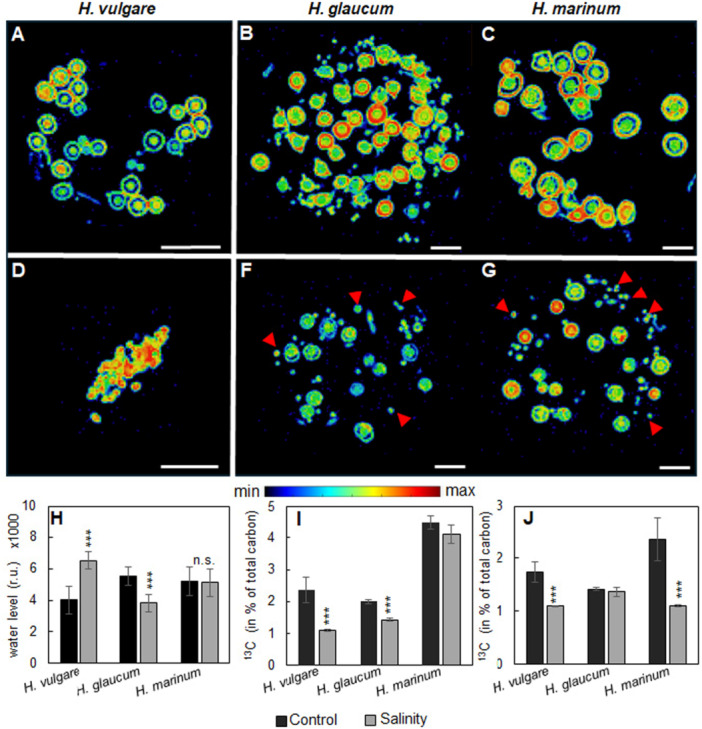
Root water distribution, and ^13^C‐uptake and distribution in plants under hydroponic control and salt‐stressed conditions. (A–G) Water distribution in virtual cross sections of control (A–C) and salt‐stressed roots (D–G) of *H. vulgare* (A, D), *H. glaucum* (B, F), and *H. marinum* (C, G) as analysed by Magnetic Resonance Imaging. Min/max values of the colour bar represent the relative water concentrations. Red triangles indicate new formed roots under salinity stress. Bars, 1 mm in (A, D), 500 µm in (B, C, F, G). (H) Quantification of water content in roots of three barley species analysed in (A–G). (I, J) ^13^C‐uptake and distribution in shoots (I) and roots (J) of three barley species under control and salinity stress conditions. Data are mean ± SD; *n* = 7–25 in (H), *n* = 3–5 in (I, J), *t* significant (Student's *t*‐test) at: p* < 0.001, n.s., not significant. [Color figure can be viewed at wileyonlinelibrary.com]
